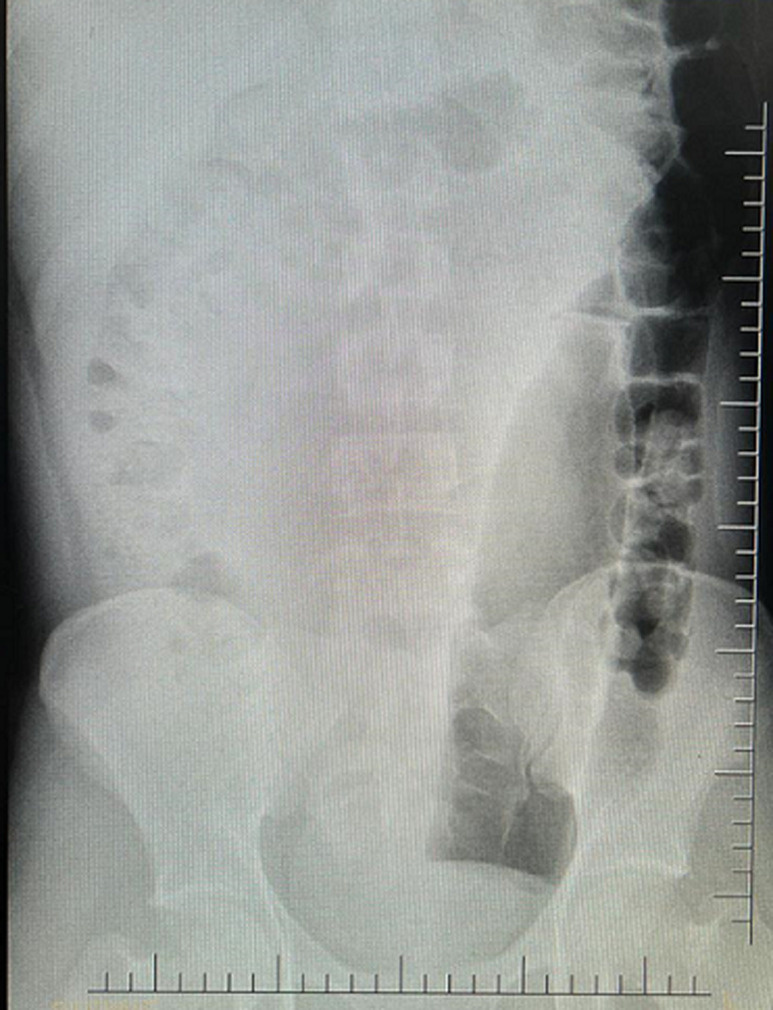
plain abdominal X-ray showing a lucent foreign body on the left side with proximal tapering
